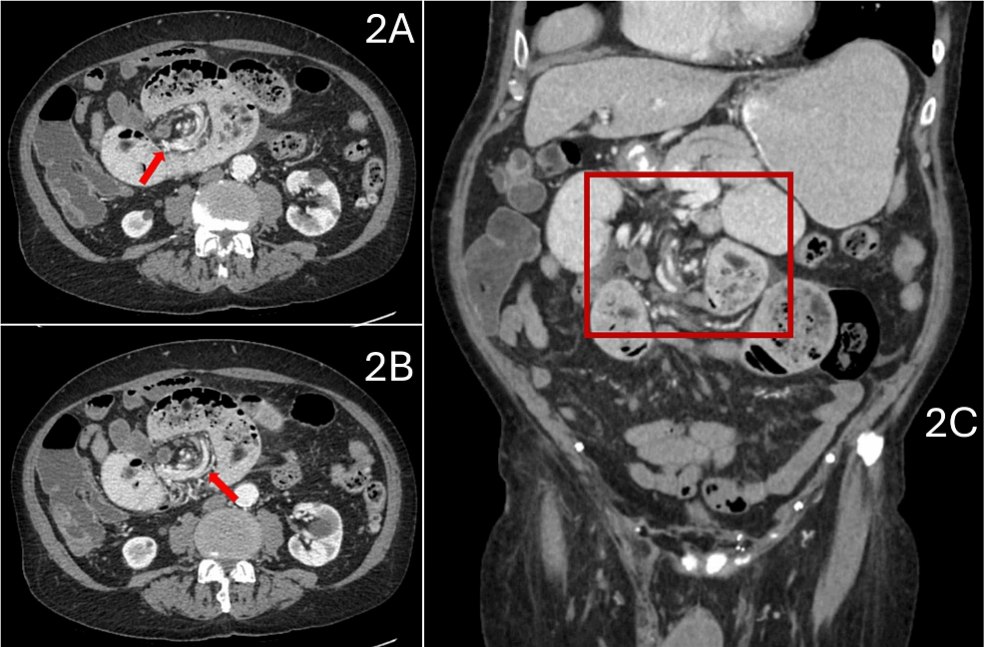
CT imaging of the abdomen and pelvis 1A and 1B: CT axial imaging of the abdomen/pelvis following oral contrast demonstrating whirring mesentery at the site of obstruction (red arrows). Significant distention of the proximal bowel loops can also be identified adjacent to the obstruction. This creates a fecalization sign that is suggestive of long-standing obstruction. 1C: CT coronal imaging of the abdomen/pelvis following oral contrast with the whirring of the mesentery at the site of the obstruction (red box). Noticeable distal collapse of bowel loops can also be identified to evidence the diagnosis of SBO. CT: computed tomography, SBO: small bowel obstruction
